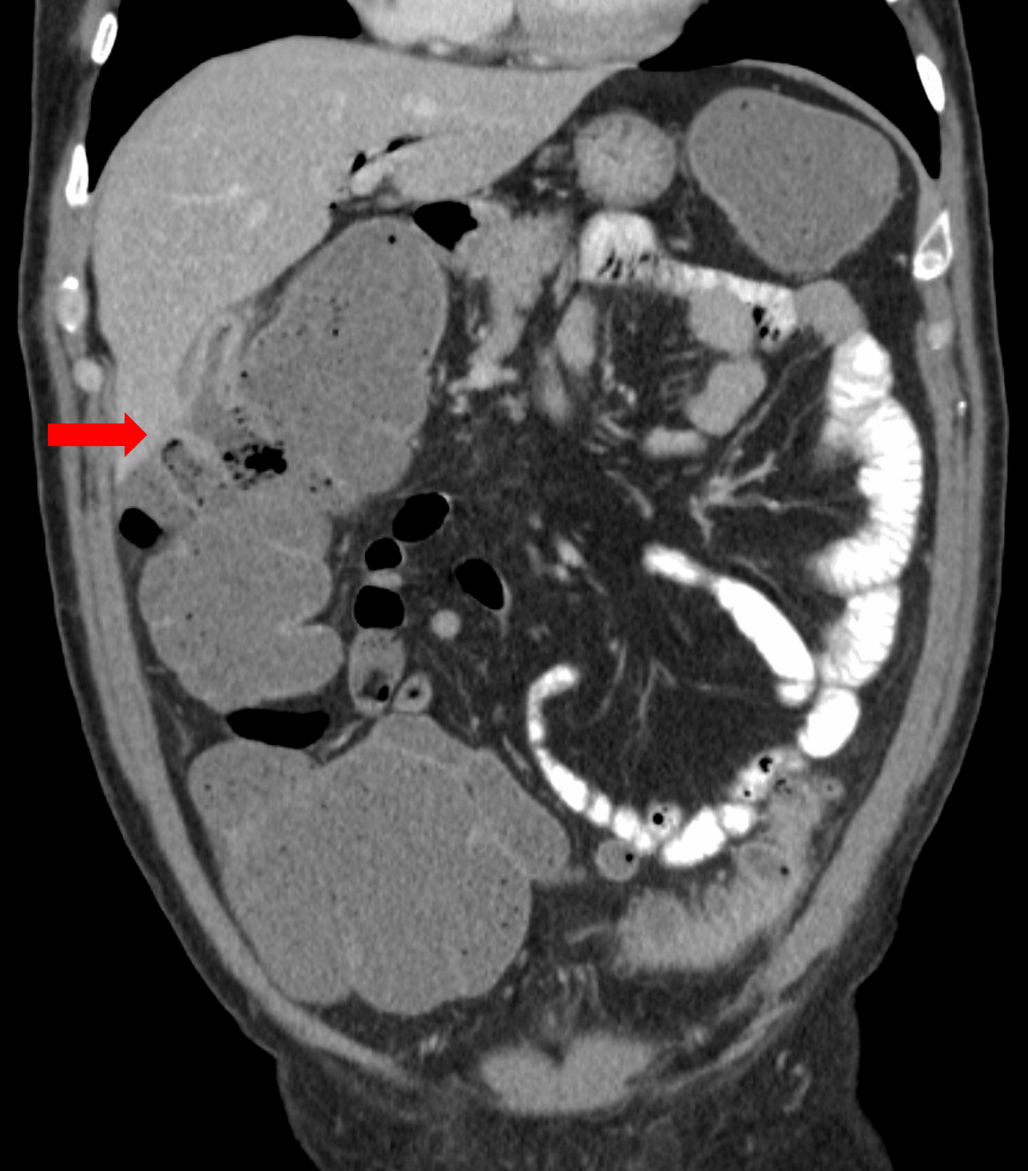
CT scan of the abdomen showing a cholecystocolonic fistula at the hepatic flexure. The red arrow indicates a cholecystocolonic fistula. CT, computed tomography
